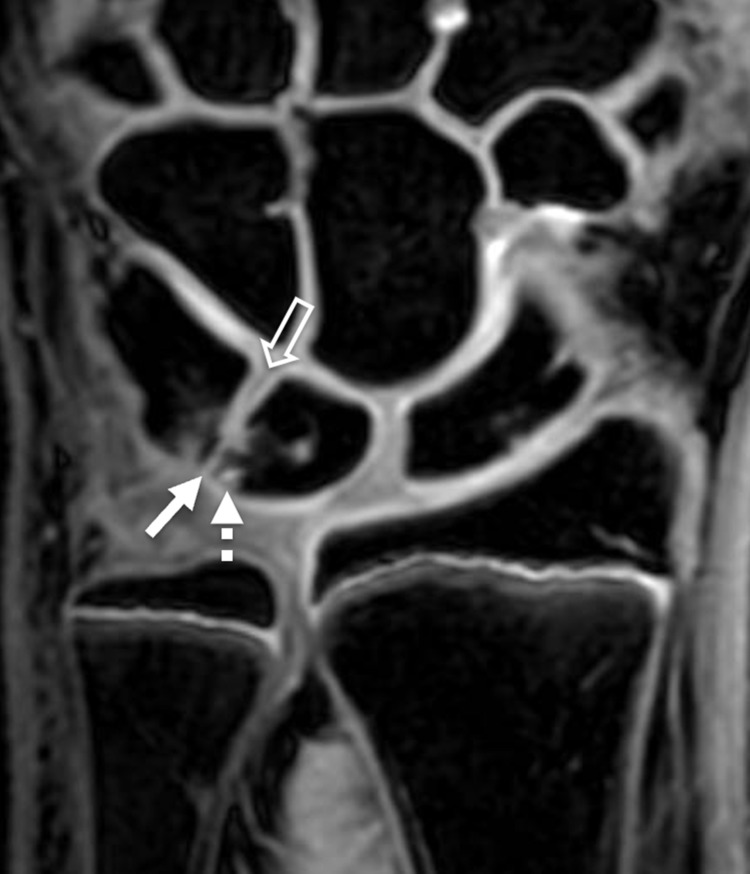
Coronal gradient echo magnetic resonance (MR) image of the left wrist in the same child The MR image shows a partial cartilaginous coalition of the proximal aspect of the lunotriquetral joint (solid arrow). Associated subchondral cystic change is also present (dashed arrow). Notice that the distal portion of the joint appears normally formed (open arrow), indicating that this is a partial coalition.
